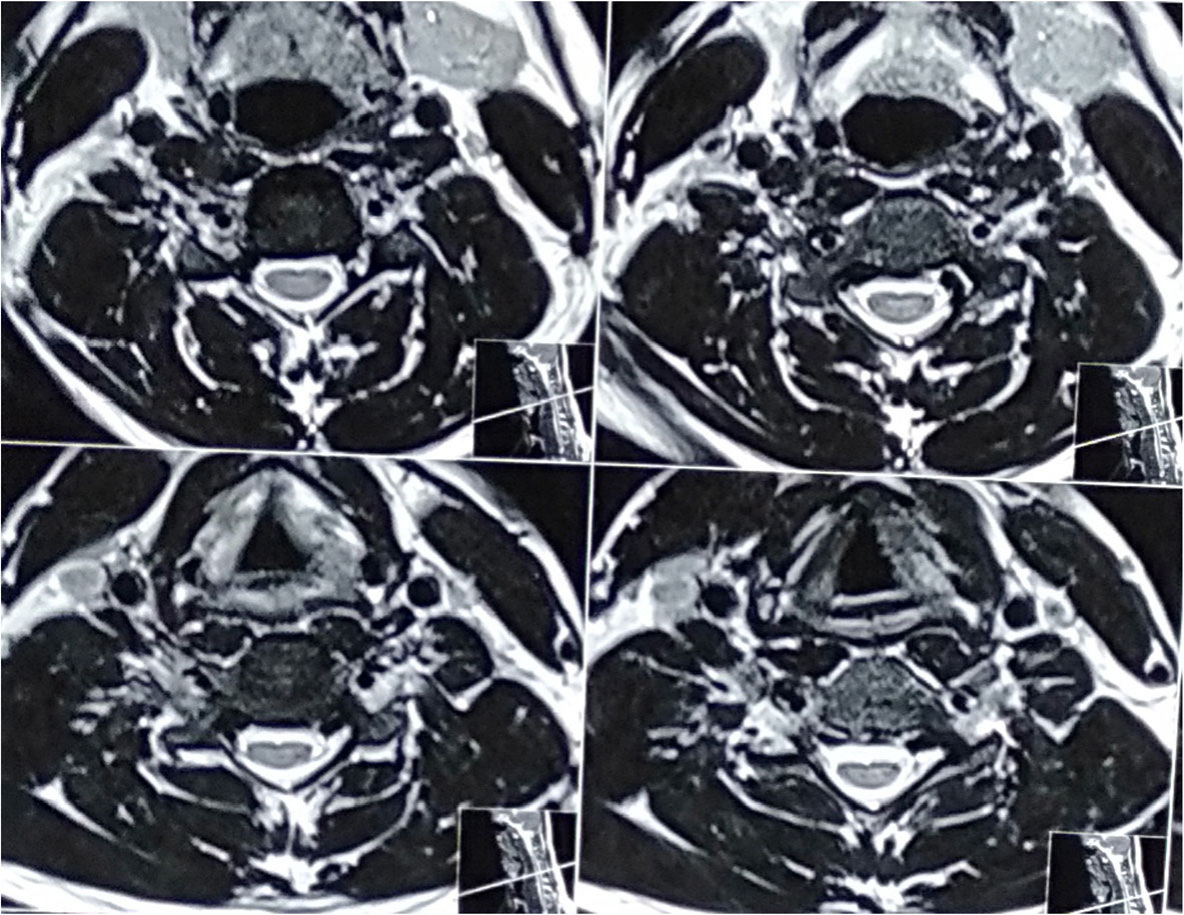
Axial T2-weighted cervical images without prominent cord hyperintensity or cord displacement
